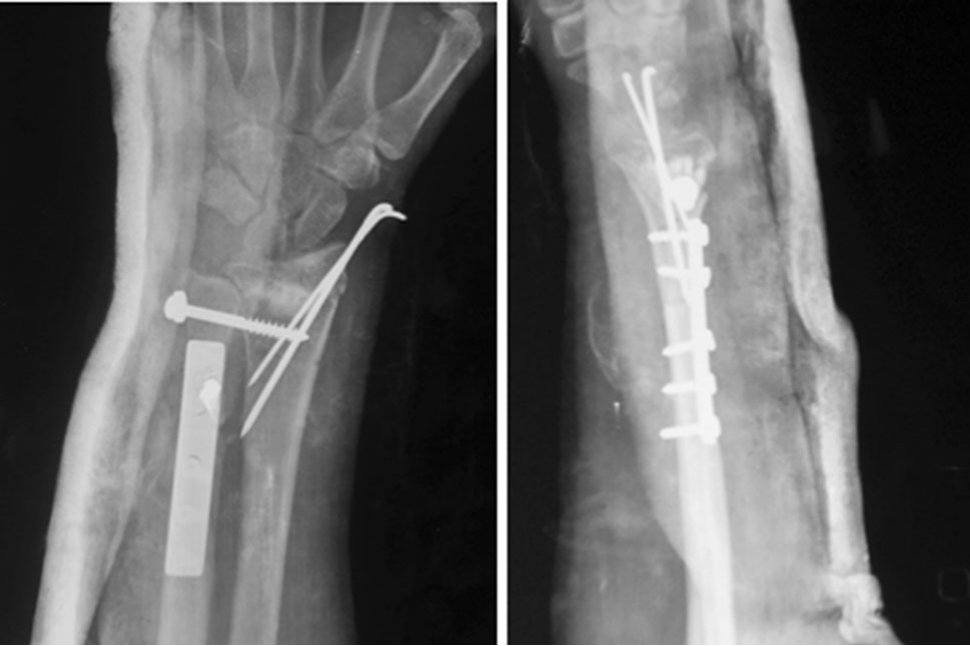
Post operative radiographs of the same patient, showing restoration of distal radius anatomy
